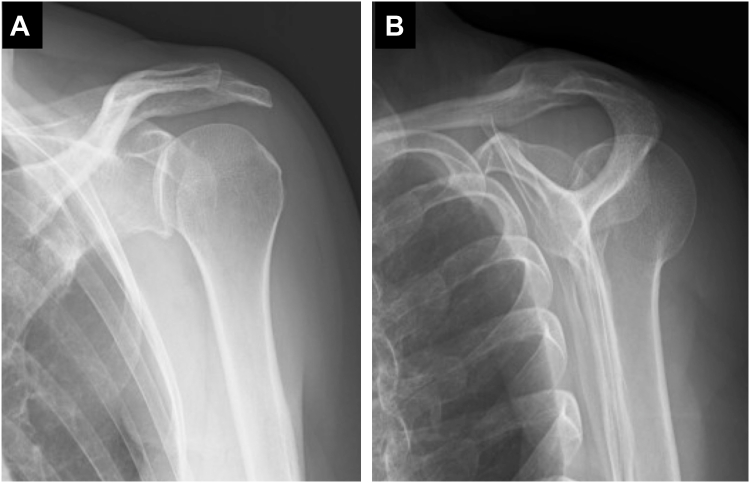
Initial radiographs of Case 1. (A) Anteroposterior view. (B) Scapula Y view.
